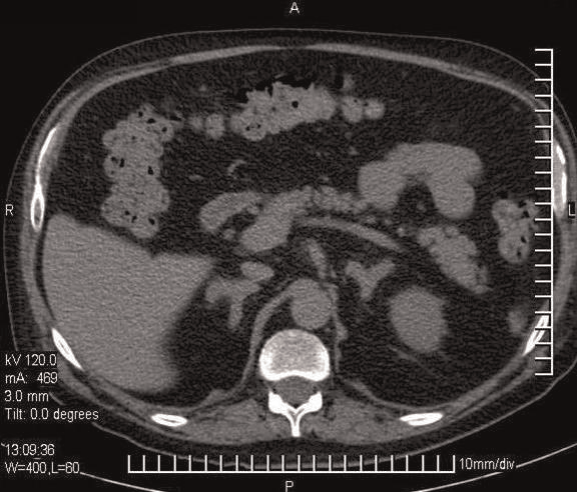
Abdominal CT scan showing diffuse enlargement (hyperplasia) of the adrenal glands without focal lesions. No metastatic lesions were observed in the liver and spleen. Also there was not lymph node enlargement.
